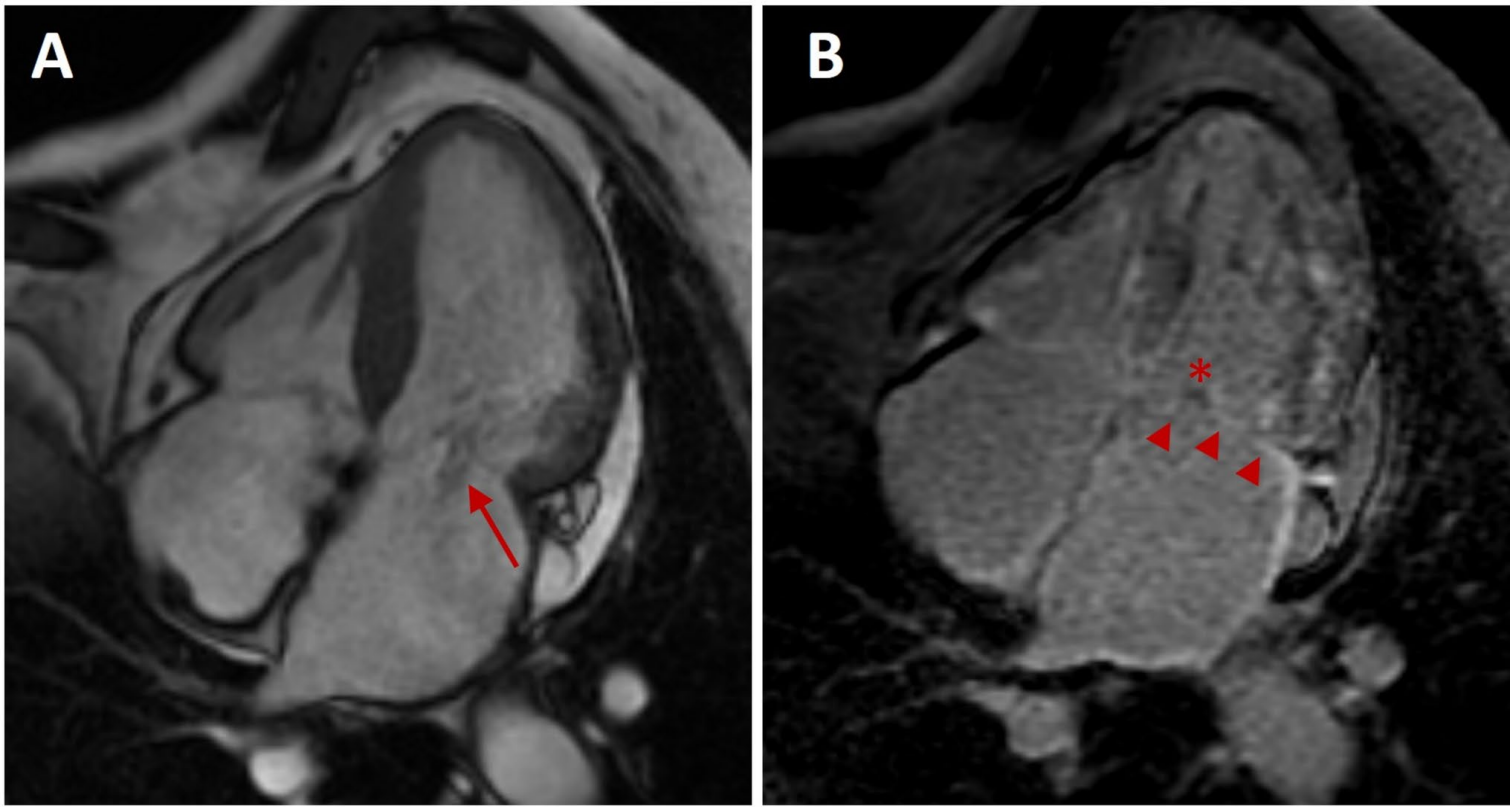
Magnetic resonance imaging in a AL-CA patient with moderate mitral regurgitation. A Cine steady-state free-precession four-chamber view in early systole, showing an increased biventricular and biatrial wall thickness, with flow turbulence (*red arrow*) due to mitral regurgitation. B Late gadolinium four-chamber view, showing biventricular and biatrial enhancement consistent with amyloid deposition, more prominent in the subendocardim; note late enhancement of the mitral leaflets (*red arrowheads*), with some very small hypointense areas due to calcifications (*asterisk*)
